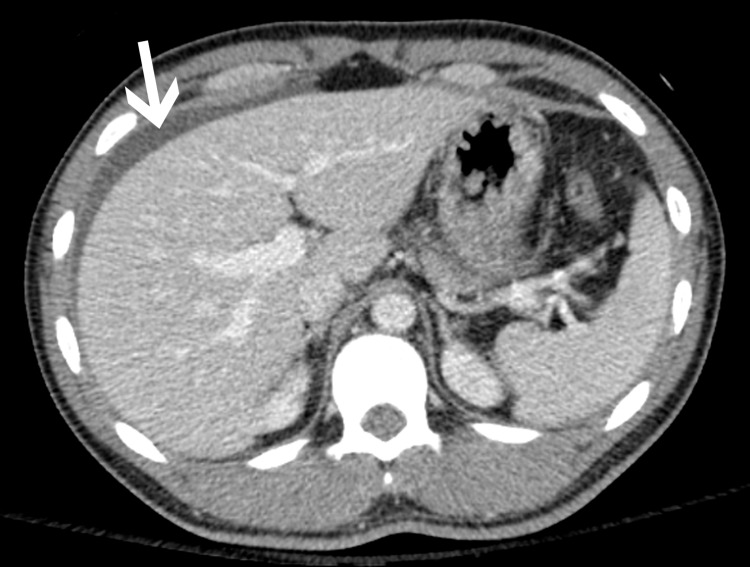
Axial CT image through the upper abdomen shows ascites (white arrow) adjacent to the liver.
